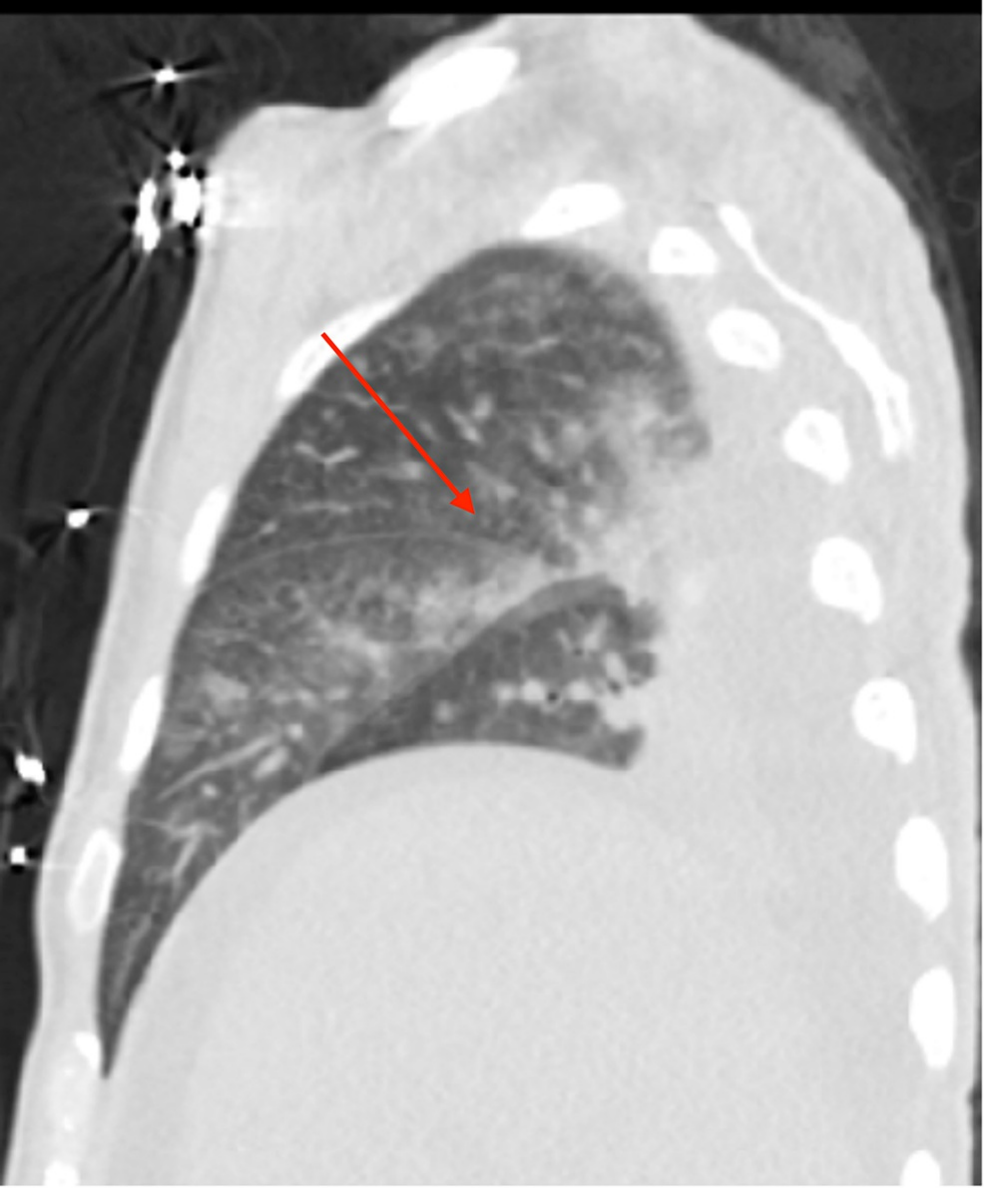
CT scan of the chest revealing patch ground-glass consolidation
